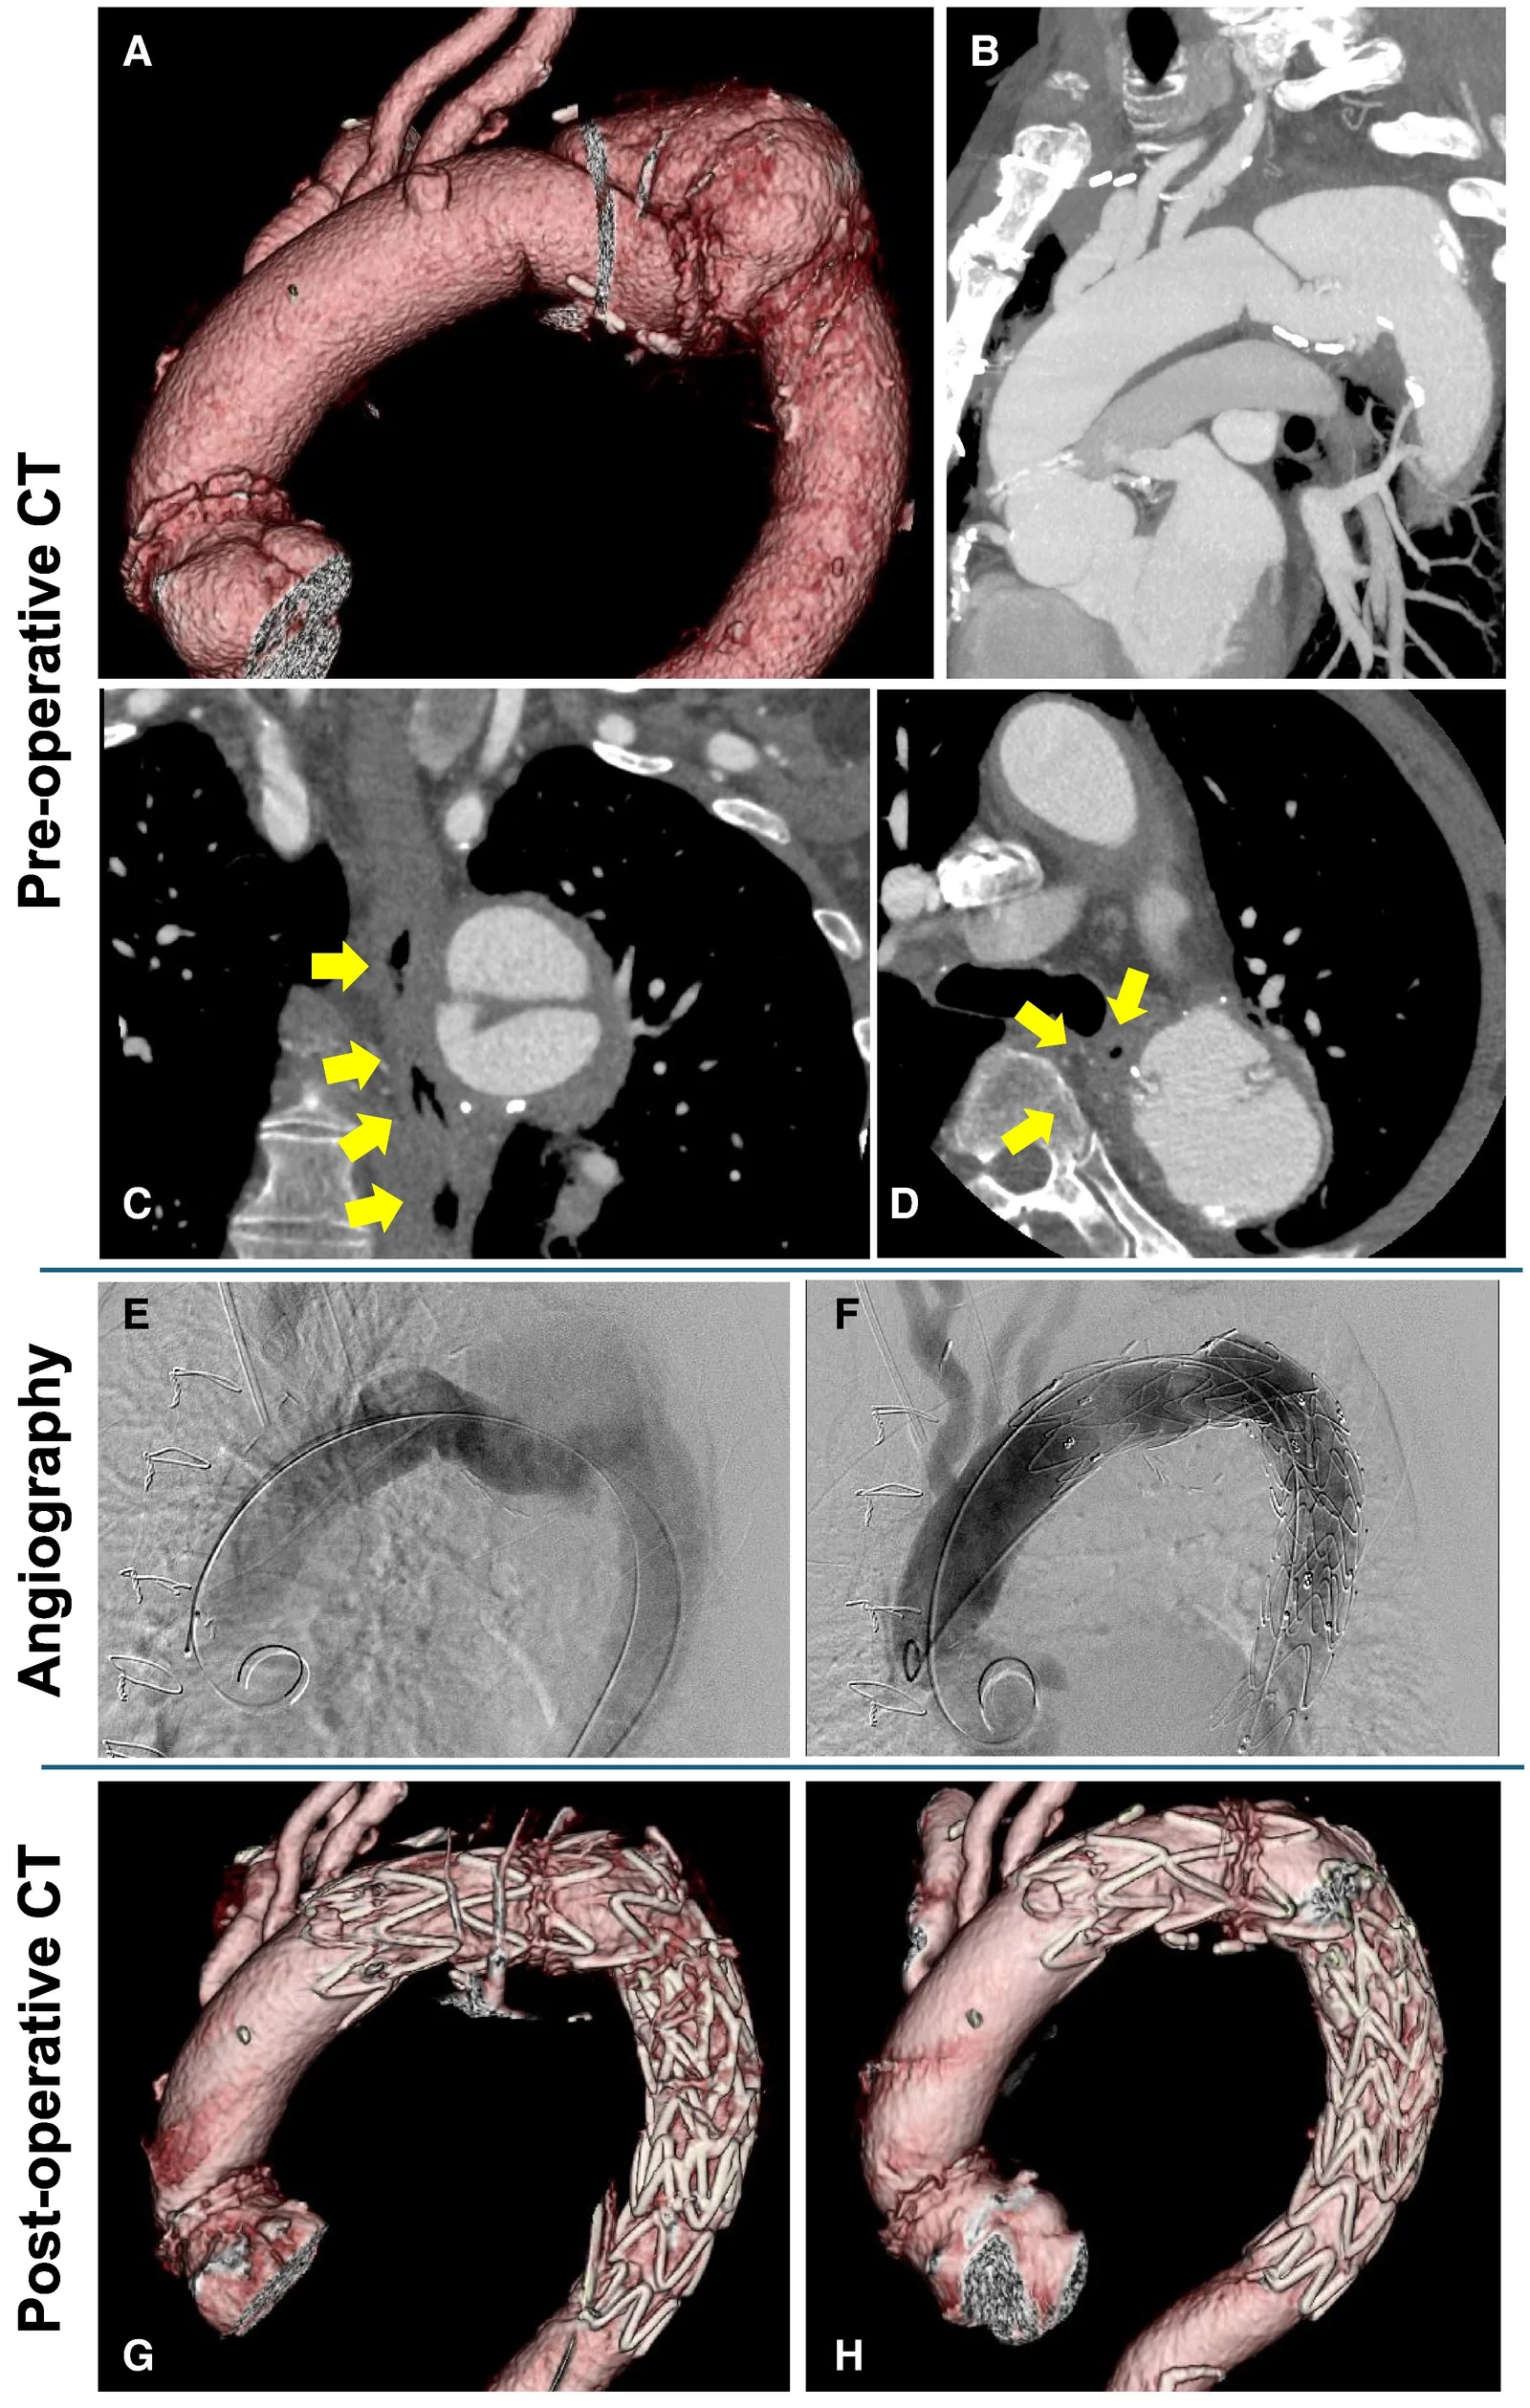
Computed tomography and aortography images. (*A–D*) Pre-operative computed tomography images showing a pseudoaneurysm measuring ∼7 cm at the distal anastomosis, with compression of the oesophagus (yellow arrows in *C* and *D*). (*E*) Peri-operative aortography demonstrating no extravasation outside the pseudoaneurysm. (*F*) Successful deployment of the stent grafts. (*G* and *H*) Post-operative CT following thoracic endovascular aortic repair confirming the absence of contrast leakage at 1 week (*G*) and 2 weeks (*H*). CT, computed tomography; TEVAR, thoracic endovascular aortic repair.
